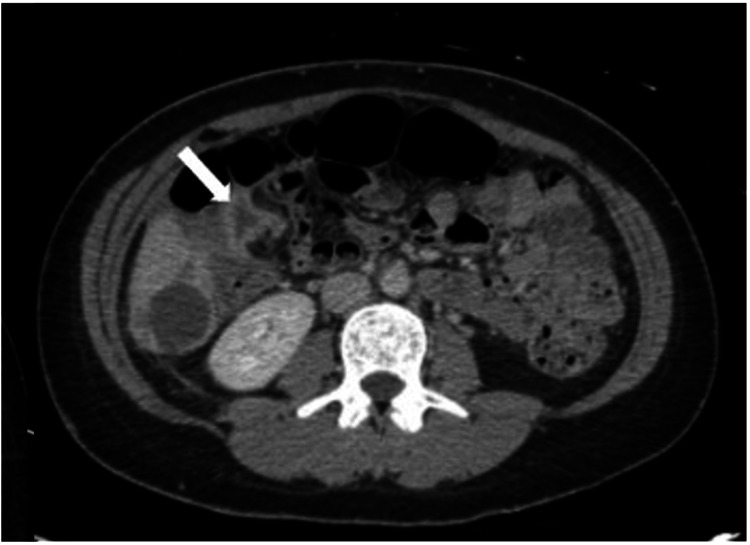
Computed tomography shows medial right pericolonic fluid collection Axial computed tomography (CT) from postoperative day 17 showing a 2.2 x 1.8 cm collapsed fluid collection involving the wall of the medial right colon.
